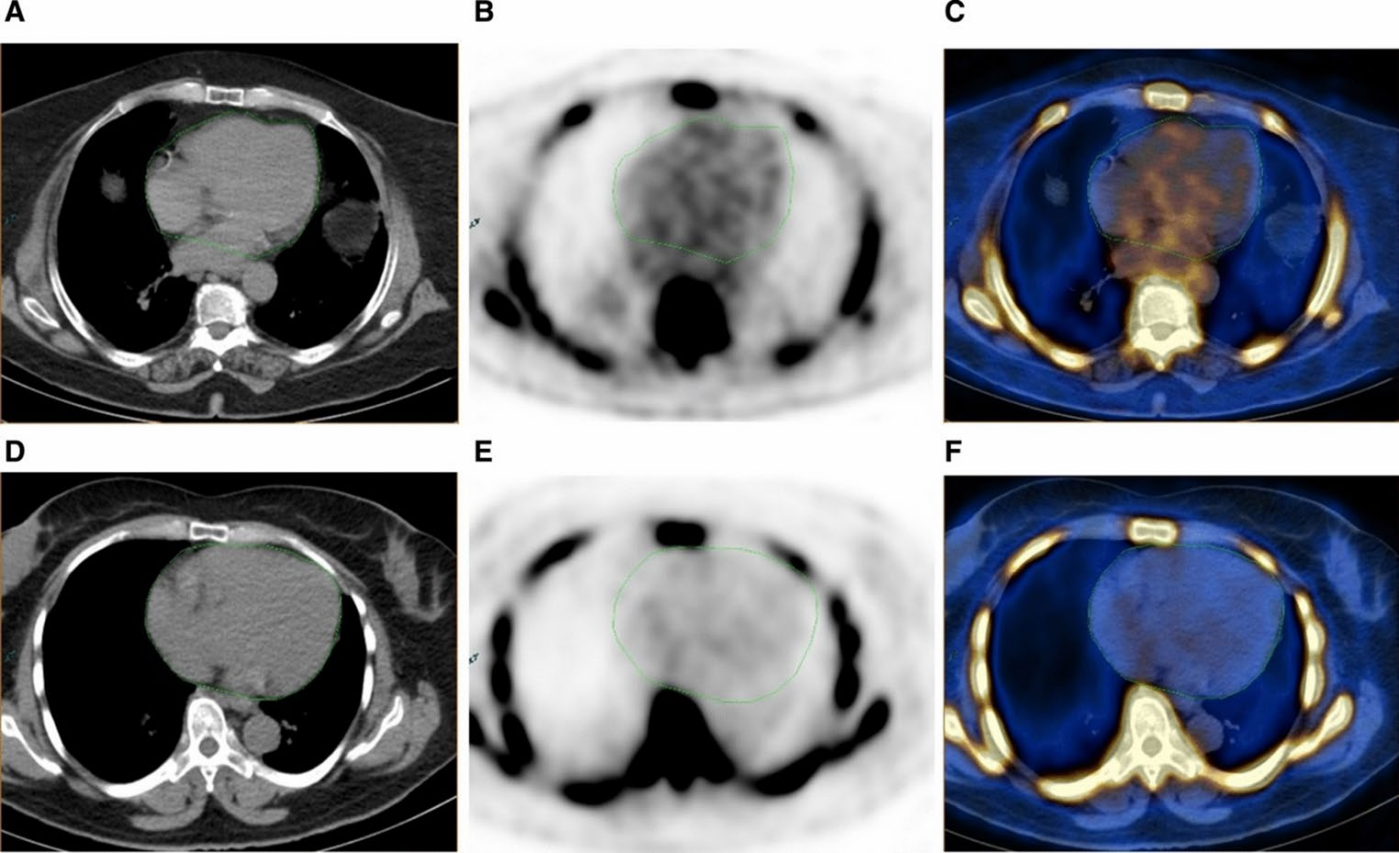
Subject with high global molecular calcium score (GMCS) (548) 18 F-NaF heart uptake (top) compared to subject with low GMCS (291) 18 F-NaF heart uptake (bottom). The patient displays greater radiotracer uptake in the PET images (B and E) and PET/CT fusion images (C and F), indicated by colored pixel intensity. (Reproduced from: Borges-Rosa J, J Nucl Cardiol Off Publ Am Soc Nucl Cardiol. 2022 Aug;29 [4]:1846–54, with permission from Elsevier) [32]
